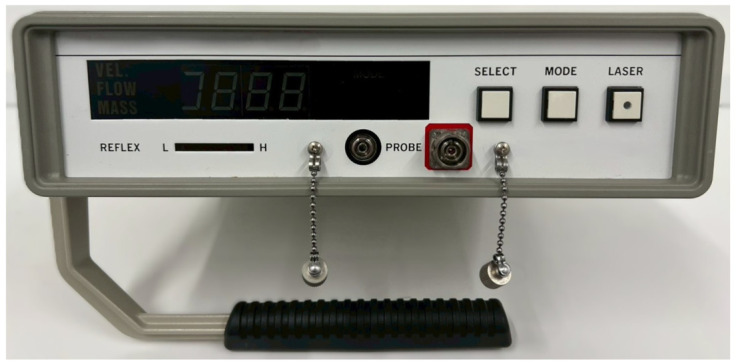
Laser Doppler flowmeter used for measuring gingival blood flow.
